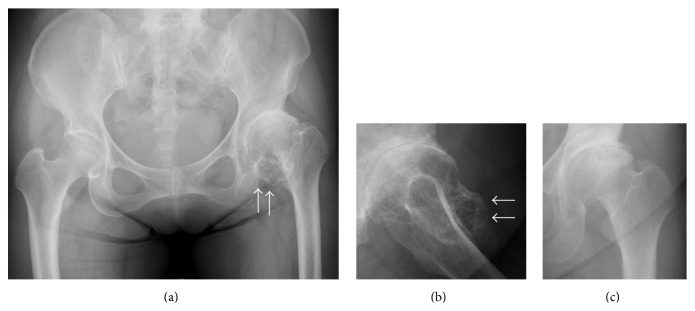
Radiographs of the left hip. Anteroposterior (a) and lateral (b) views of the left hip at initial presentation. The osseous mass (white arrows) appeared to have a connection to the femoral head on the mediolateral view of the X-ray. Anteroposterior X-ray obtained 8 years earlier (c). No abnormal bone mass is observed.
